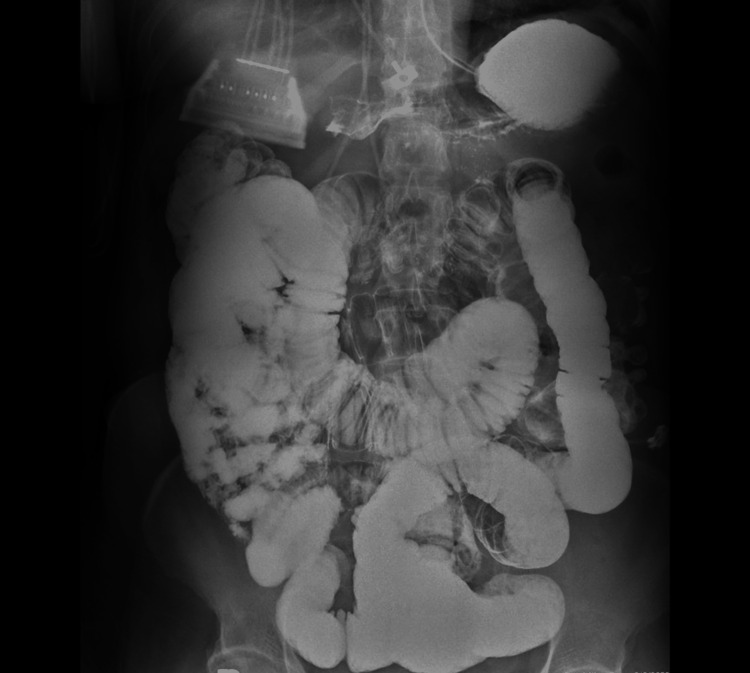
Upright KUB X-ray hospital day three KUB: kidney, ureter, and bladder
